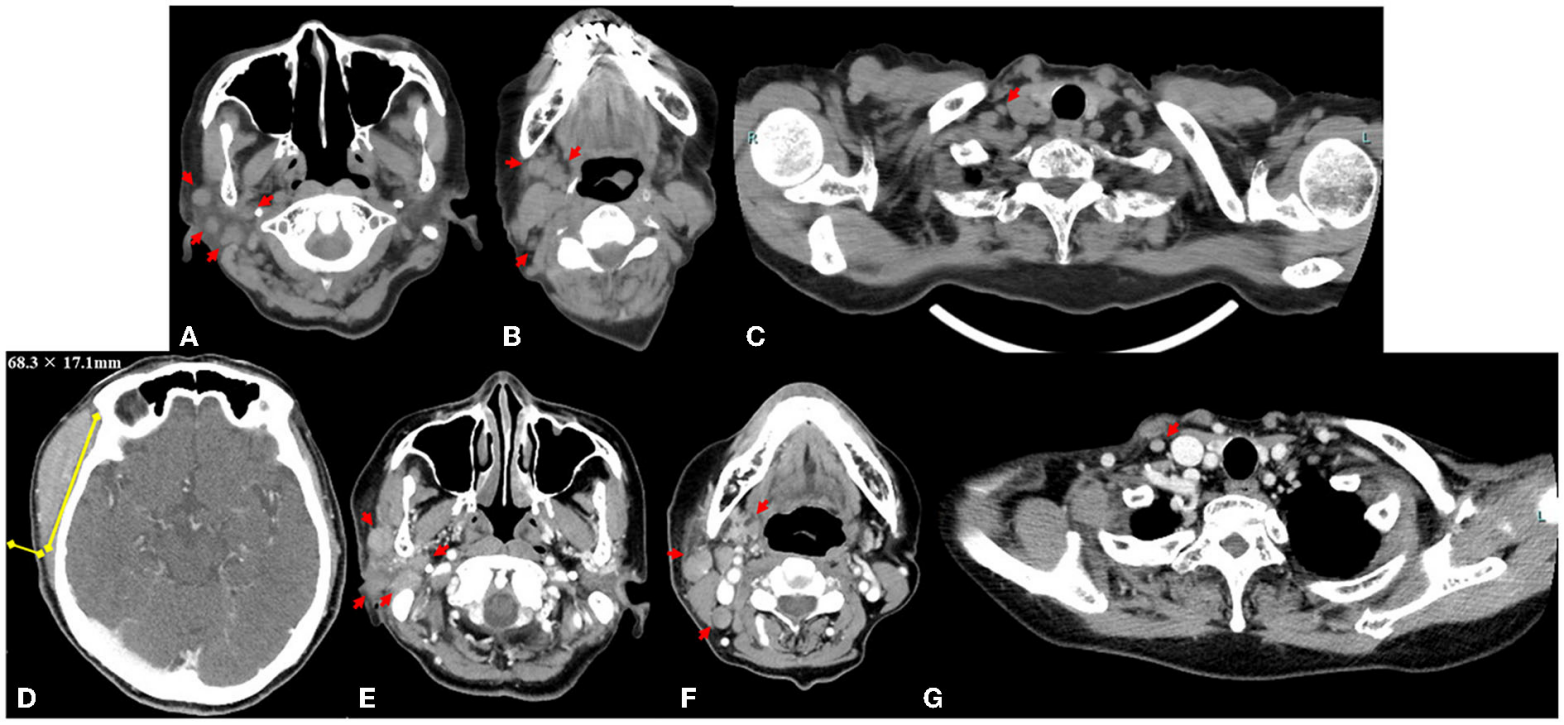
Axial computed tomography image obtained at the first visit shows 14 lymphadenopathies, with a maximum diameter ≤ 7.5 mm (red arrows) in the right parotid region (A), submandibular and jugular regions (B), and supraclavicular region (C). Measurement of the right temporal mass (68.3 × 17.1 mm) on an axial contrast-enhanced computed tomography image 9 weeks after the first visit to our institution (D). The lymphadenopathies (maximum size ≤ 13.3 mm, yellow arrows) in the right parotid region (E), submandibular and jugular region (F), and supraclavicular region (G).
